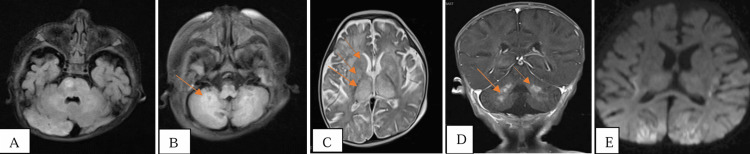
MRI brain – plain and contrast images A and B: Fluid-attenuated inversion recovery (FLAIR) axial section showing brainstem and cerebellar peduncle hyperintensities; C: T2 axial section showing basal ganglia and thalamic hyperintensities and subcortical white matter hyperintensity; D: T1 contrast axial section showing nodular contrast enhancement in cerebellar white matter; E: Diffusion-weighted image showing restricted diffusion in the bilateral occipital and insular cortex.
